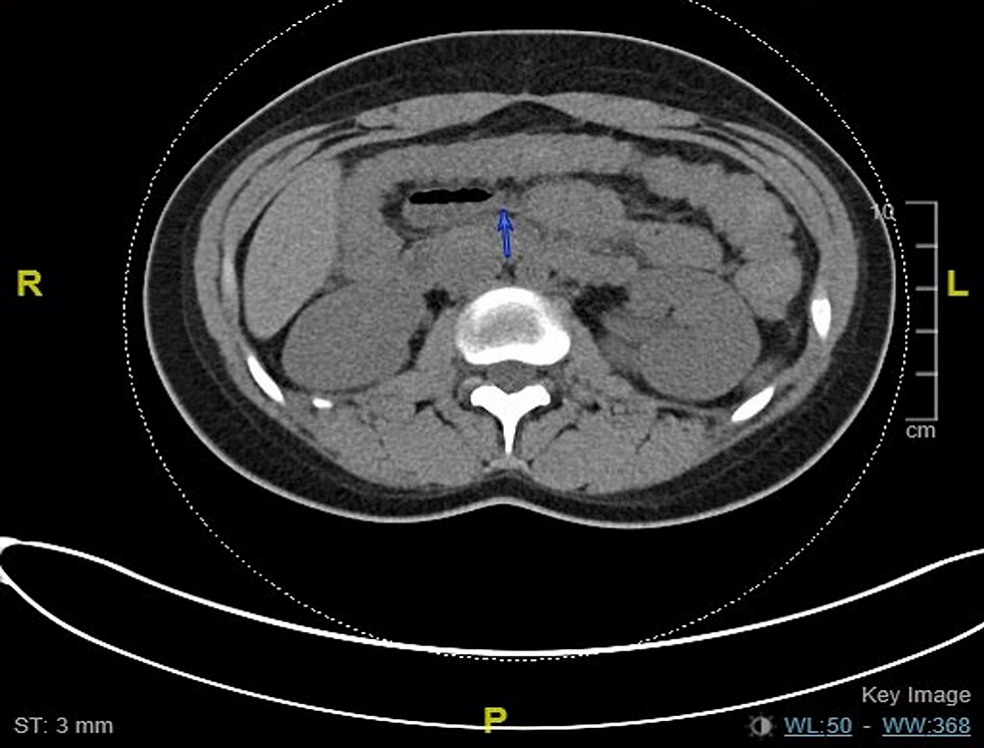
CT abdomen with the first transition point
